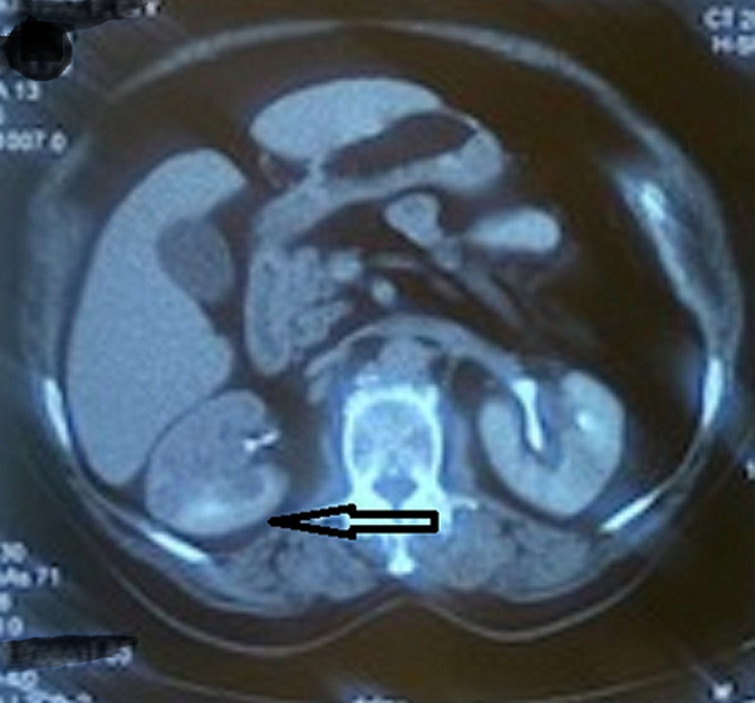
abdominal computed tomography (CT) scan demonstrates a mass in the right renal pelvis (arrow)
